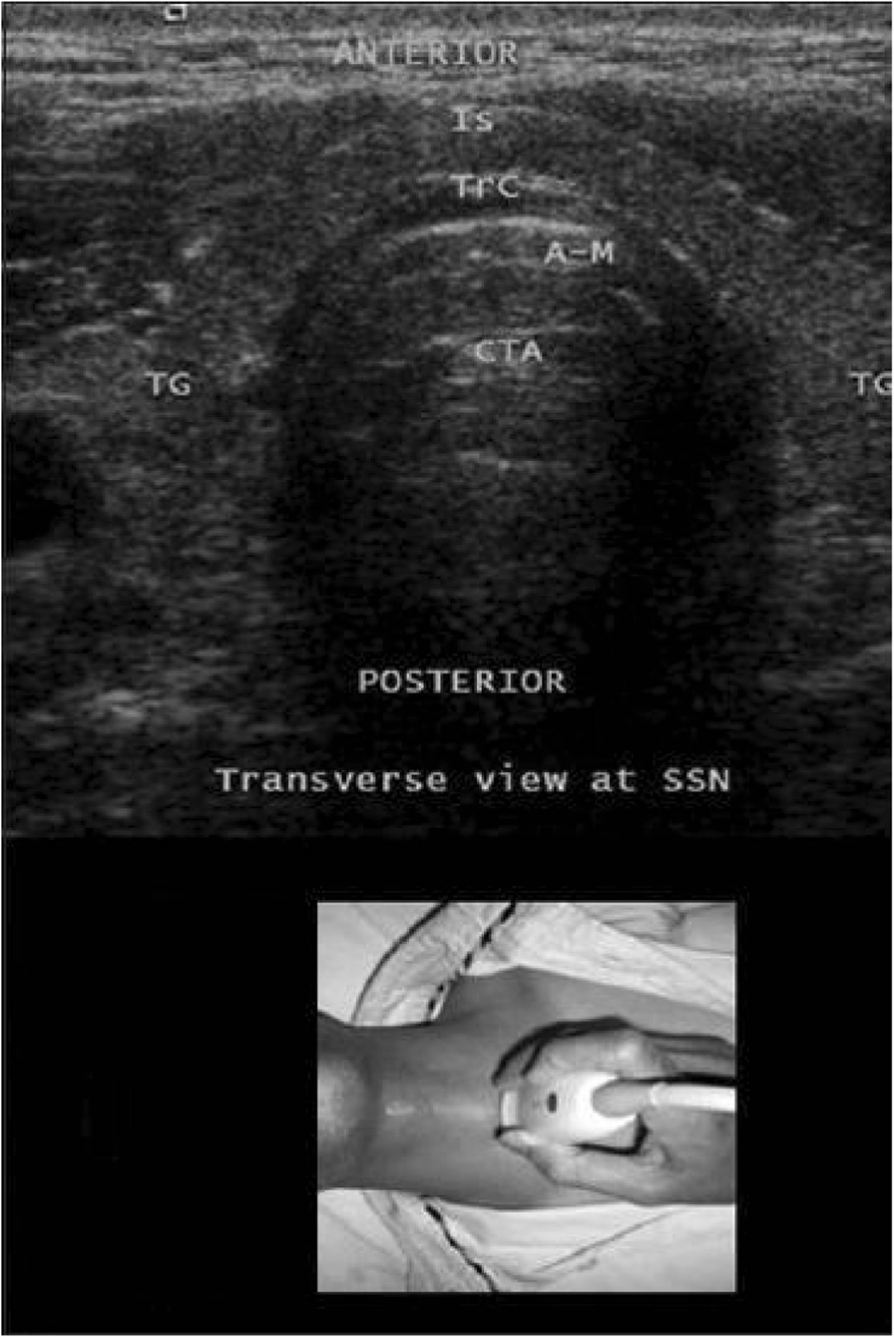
Transverse view at the level of the suprasternal notch (SSN) showing the trachea and the thyroid gland. A-M, air-mucosal interface; CTA, comet tail artefact; Is, isthmus; TG, thyroid gland; Trc, tracheal cartilage (ring). Reprinted with permission from Wolters Kluwer [42]
